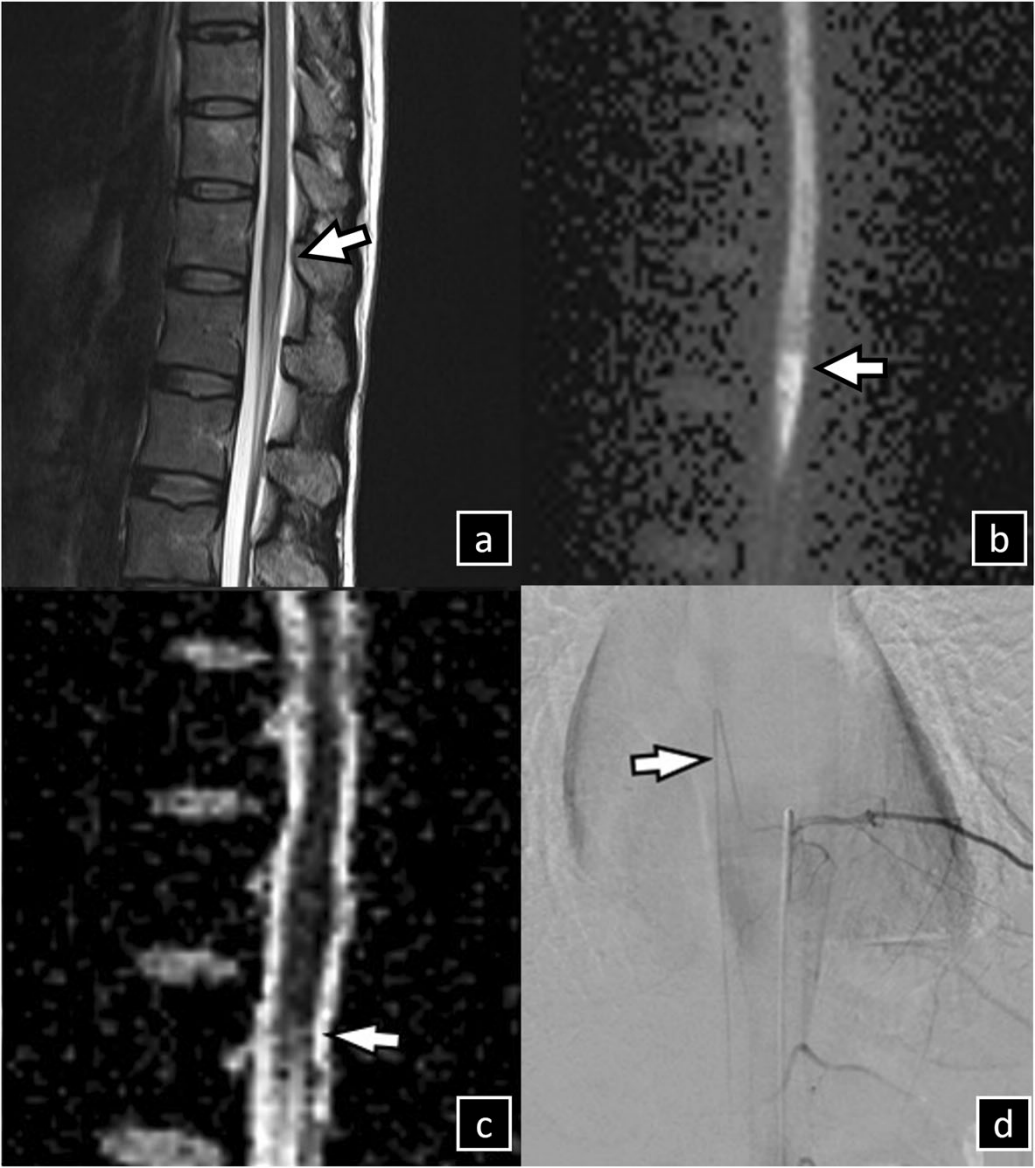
Spine images of patient 2. MRI, performed on day 2 following onset, shows typical hyperintensities on sagittal T2-weighted image (arrows in a) and diffusion-weighted image (arrows in b), as well as hypointensities on sagittal apparent diffusion coefficient image (arrows on c) in the conus medullaris T12. Spinal angiography revealed a patent anterior spinal artery, with the artery of Adamkiewicz arising at the left (d) T9 intercostal arteries. MRI, magnetic resonance imaging
